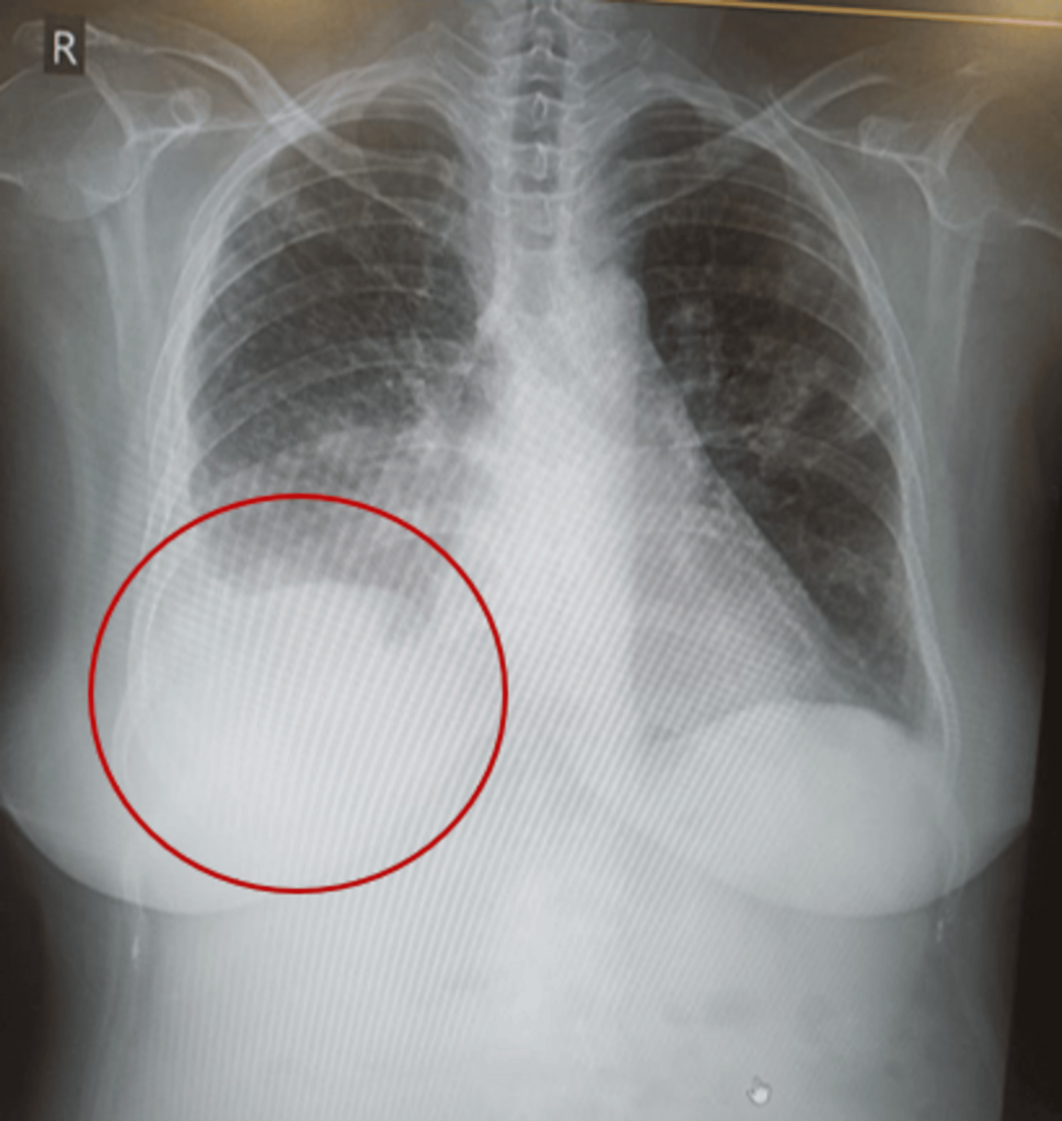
Chest X-ray showing blunted right costophrenic angle.
